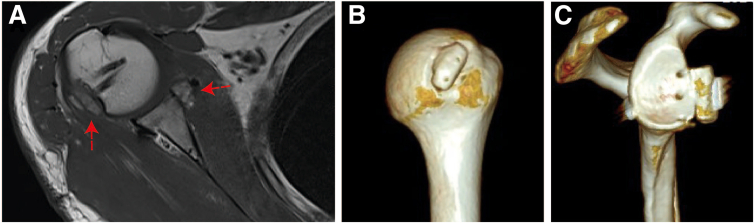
Postoperative computed tomography reconstruction and magnetic resonance imaging. The right shoulder is shown. (A) Cross-section of the shoulder on magnetic resonance imaging after surgery showing the defect filled with bone block. (B-C) Three-dimensional reconstruction of the shoulder after surgery, determined on the basis of the computed tomography scan, showing the graft.
